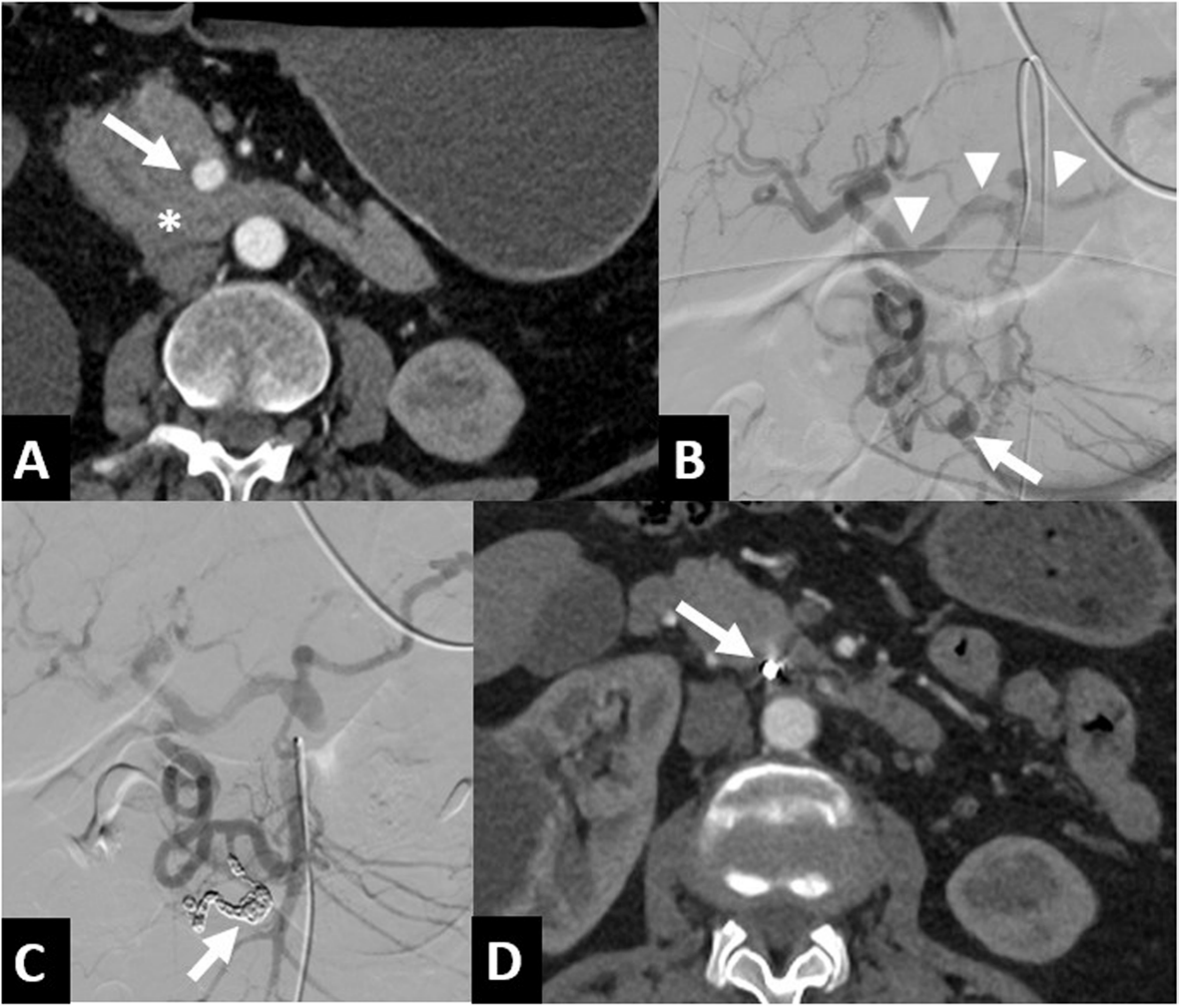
70 year-old female referred to emergency room for abdominal pain (Patient n°3). A The abdominal CT scan in axial section at arterial phase revealed a retro-portal hematoma (*) and a 12-mm saccular PIPDA aneurysm (white arrow). B Angiography of the SMA confirmed the aneurysm (white arrow) and demonstrated retrograde vascularization of CA branches (arrow head). C After occlusion and packing using 7 microcoils, the CT scan showed no complications (white arrow). D Four months later, the follow-up abdominal CT scan in axial section at arterial phase confirmed satisfactory occlusion, with no evidence of recurrence (white arrow). Abbreviations: CA, Celiac artery; CT, Computed tomography; PIPDA, Posteroinferior pancreaticoduodenal artery; SMA, Superior mesenteric artery
